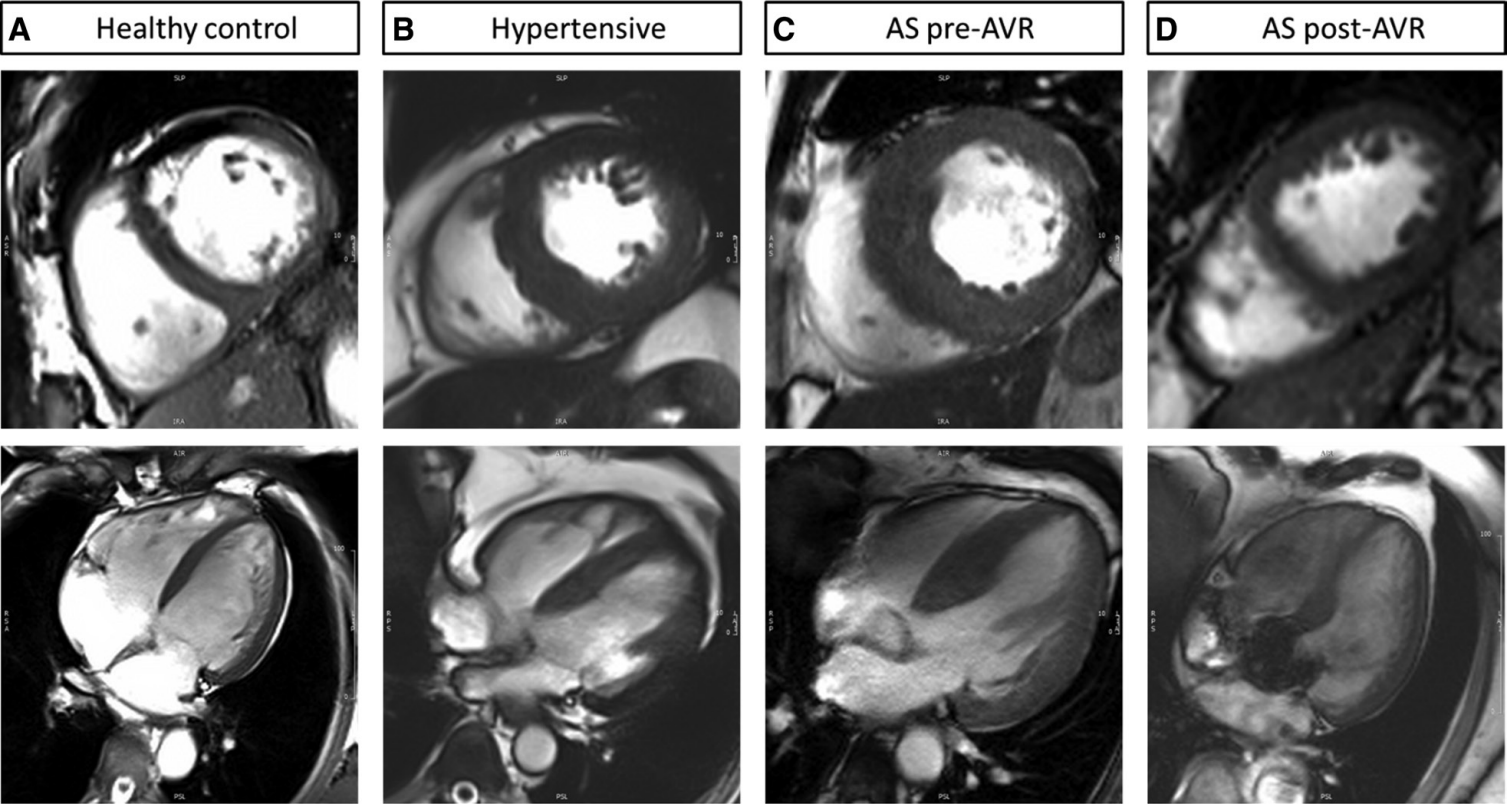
Representative short-axis and 4-chamber view of cardiac magnetic resonance images. A, Healthy control; B, patient with hypertension; C, aortic stenosis pre-aortic valve replacement (AVR); and D, post-AVR of the same patient with aortic stenosis (AS).
